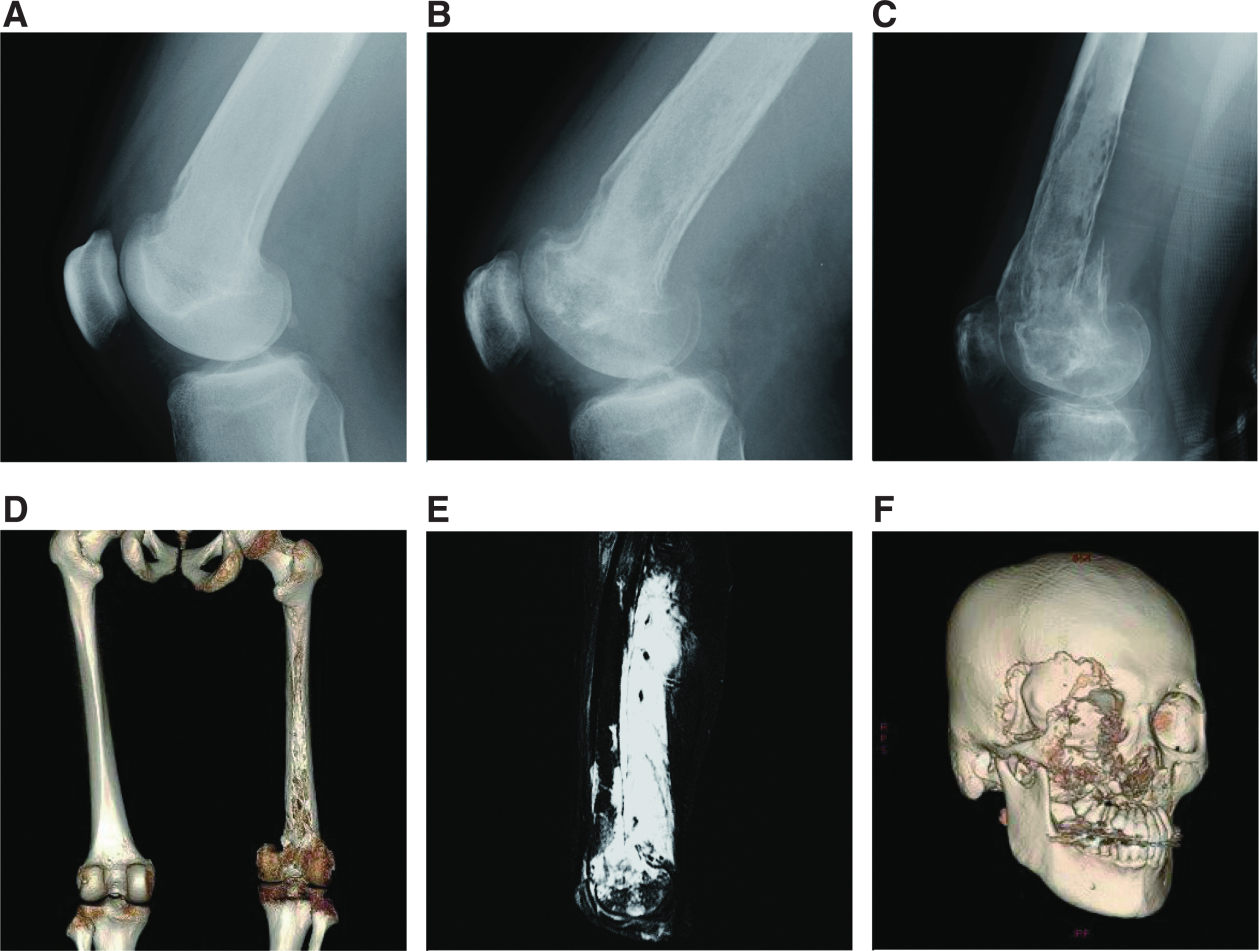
Radiological findings of patients with GSD. The osteolytic imaging of GSD patients is characterized by progressive, destructive cortical resorption that can extend beyond adjacent joints. (A–E) A 19-year-old male with GSD involving the right femur. The patient suffered from pain of the right femur, for which no specific cause was evident. (A) At onset, a plain radiograph showed osteolysis at the front of the neck of the femur. (B) Three months later, the osteolytic lesion had progressed markedly. (C–E) Three more months later, the lesion showed pathological fractures. Almost complete resorption of the right femur is shown in posterior view of three-dimensional CT imaging. High signal intensity in the soft tissue surrounding the femur was shown in sagittal fat-saturated T2-weighted MRI, as well as subcutaneous fat tissue. (F) A 41-year-old male with GSD involving the right maxillofacial region in the maxilla, the frontal bone, the temporal bone, and the zygomatic bone. The patient complained of sudden vertigo, dysphagia, and left-sided paresthesia. The patient developed skull base osteomyelitis; lateral medullary syndrome resulted from progressive osteolysis and the patient died of associated brainstem stroke. CT, computed tomography; MRI, magnetic resonance imaging.
